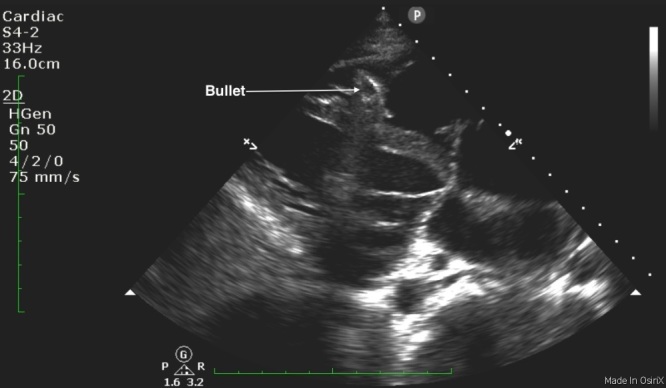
Parasternal long axis view demonstrating a bullet fragment within the right ventricle.
